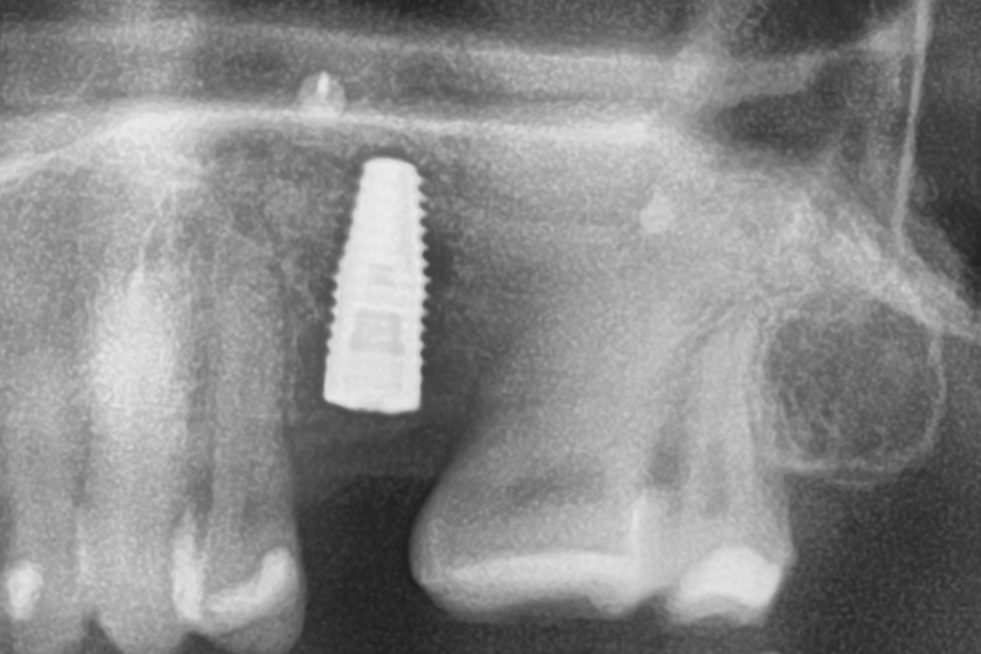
Postoperative radiograph after insertion of the implant
